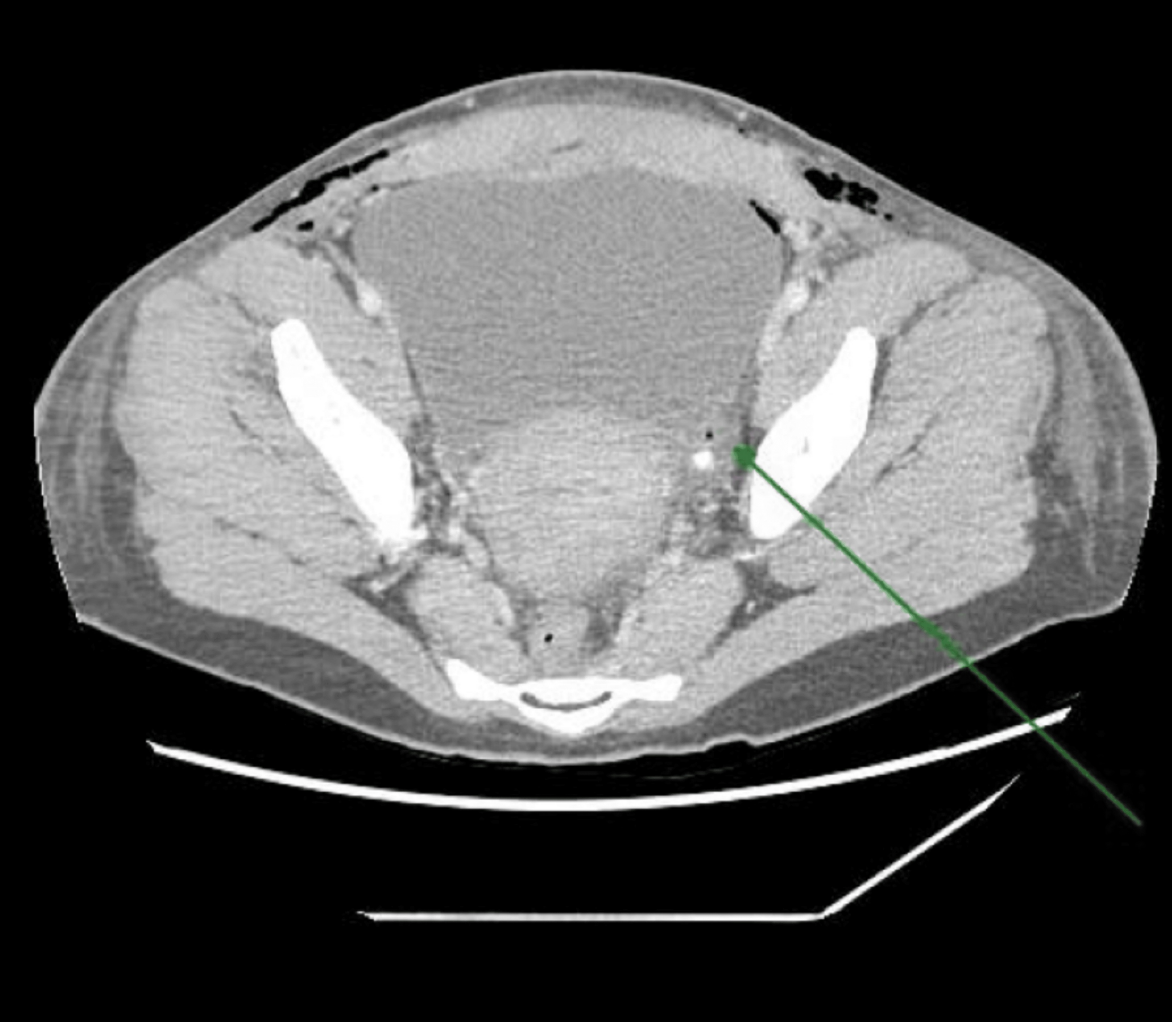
Computed tomography (CT) transverse
